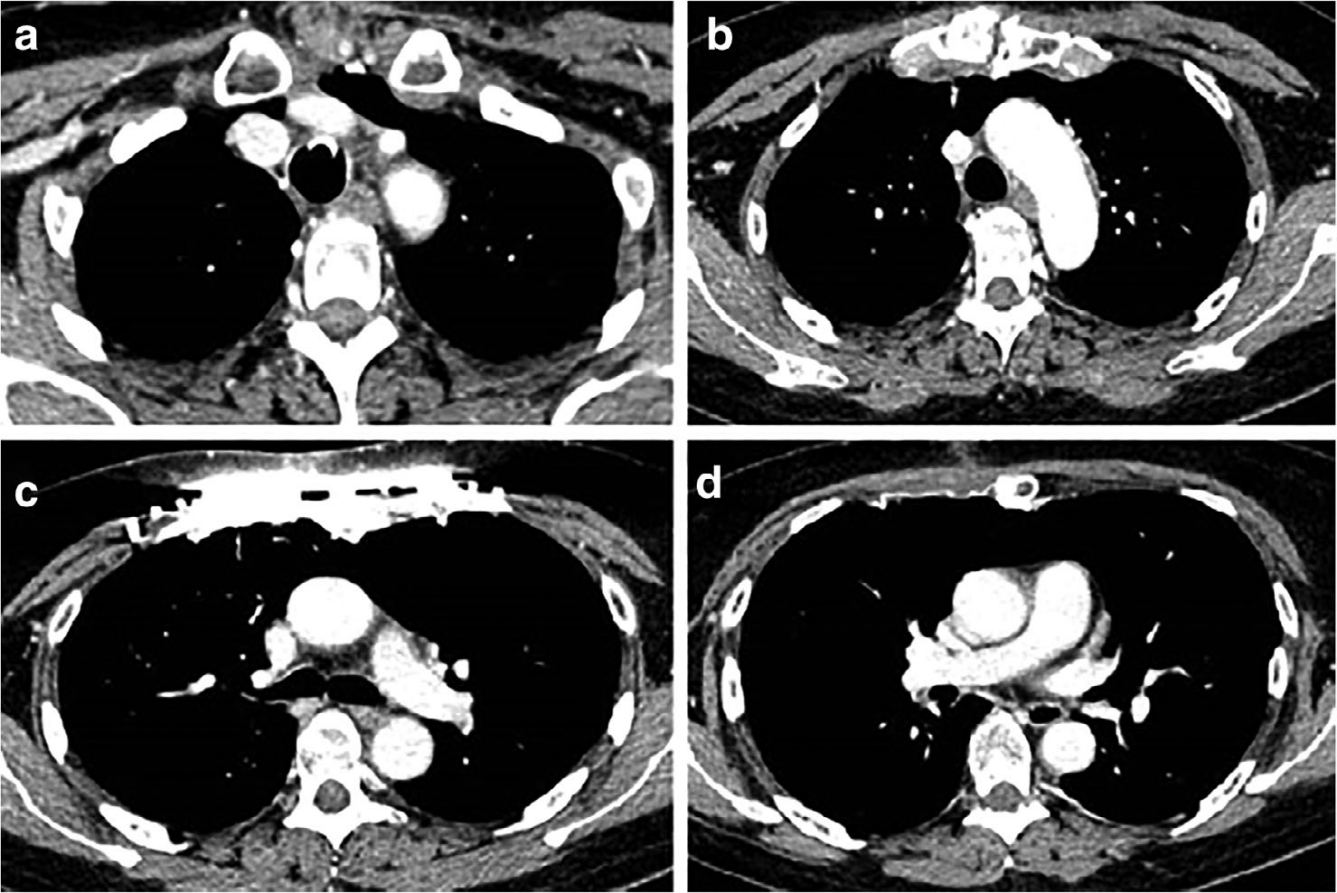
Postoperative axial view chest CT.
